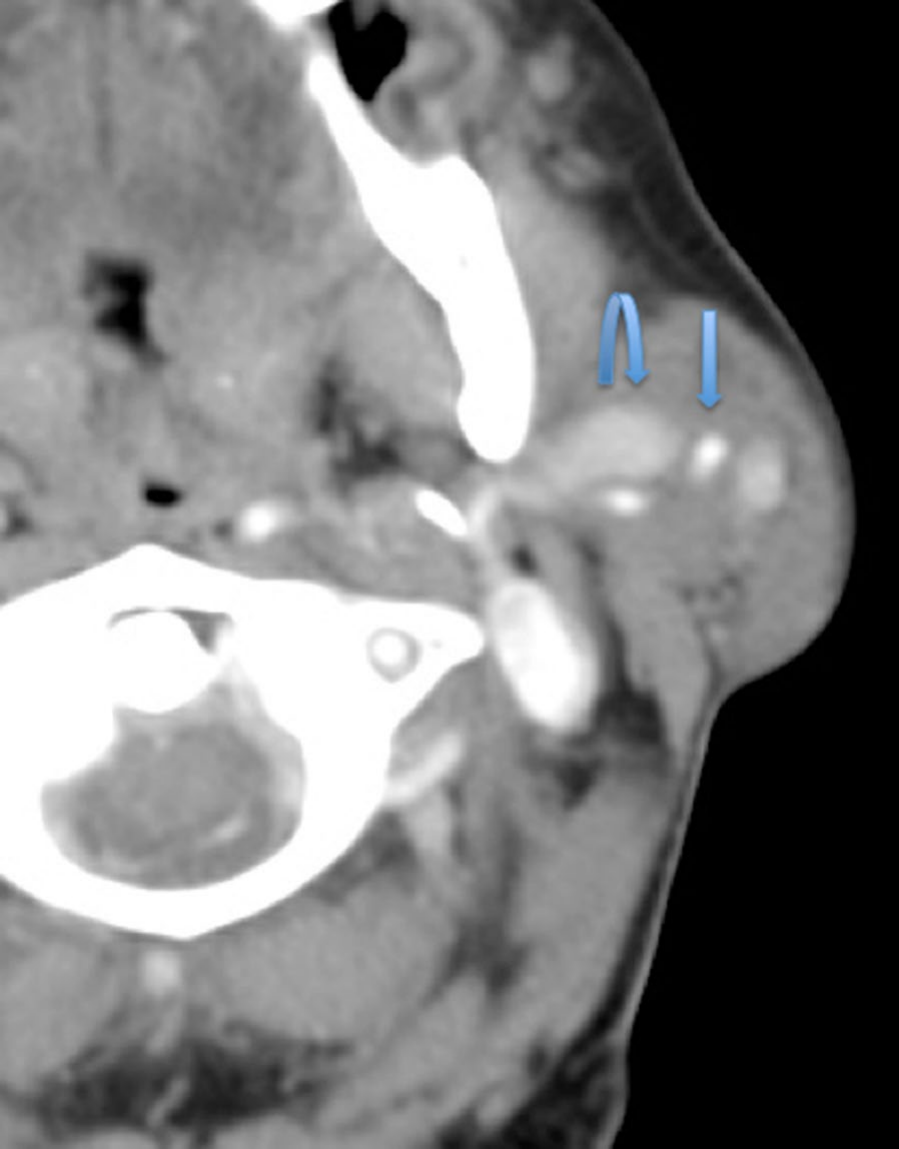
Axial contrast enhanced CT at the level of C1. Left parotid arteriovenous malformation with a left external carotid artery branch feeding vessel (*straight arrow*), along with an enlarged draining vein (*curved arrow*)
